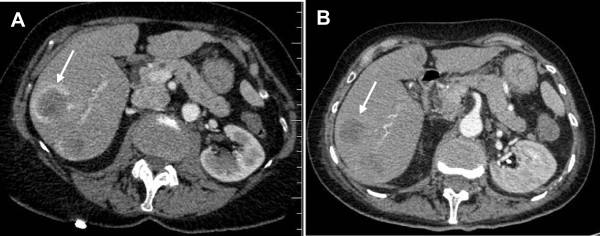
Computed tomography. The figures shows the initial CT scan of the liver (performed at the time that the metastatic stage of the disease was diagnosed) (A) and a CT scan acquired 4 weeks after the initiation of vemurafenib treatment (B). The metastasis is indicated by an arrow. Comparison of the two scans reveals a partial regression of the metastatic tumor.
